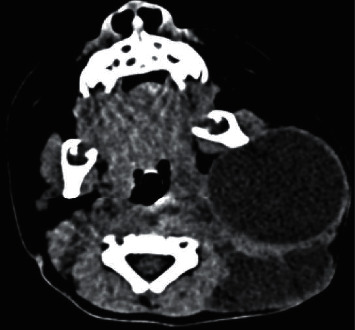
Axial computed tomography showing left cervical cystic hygroma.
